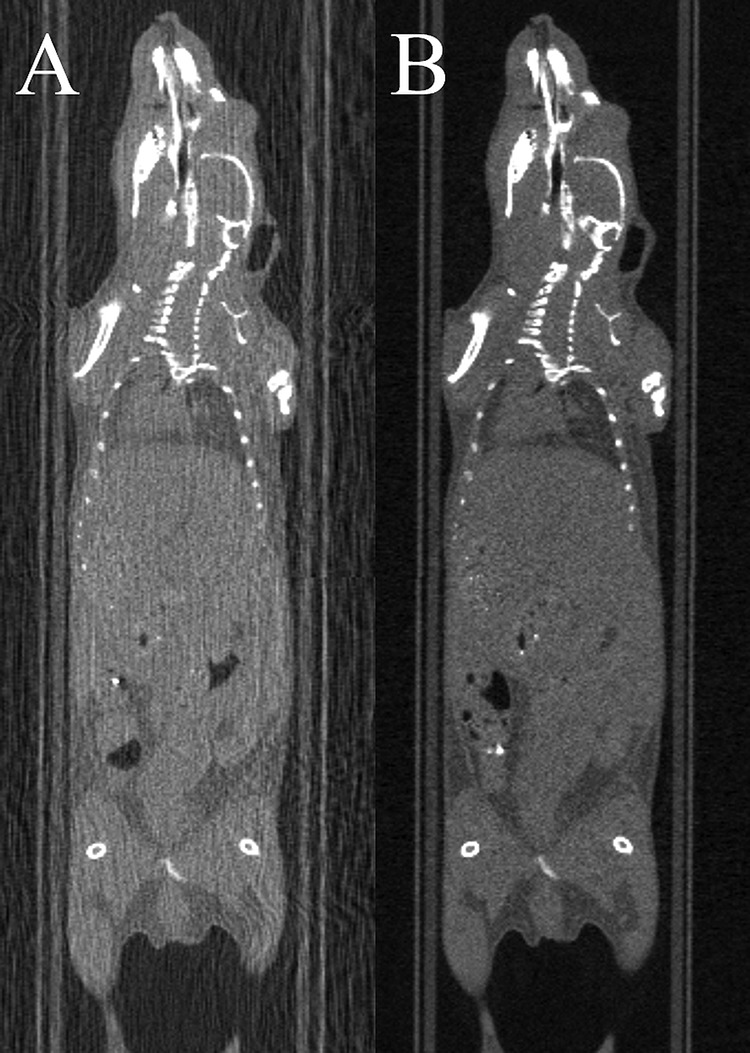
Impact of the heater material on computed tomography (CT) image quality. CT imaging using the 150-µm-diameter copper wire heater (A) or the carbon-fiber sheet heater (B). Streak artifacts owing to the presence of the heater are absent when using the carbon-fiber heater, and the image intensities remain intact.
